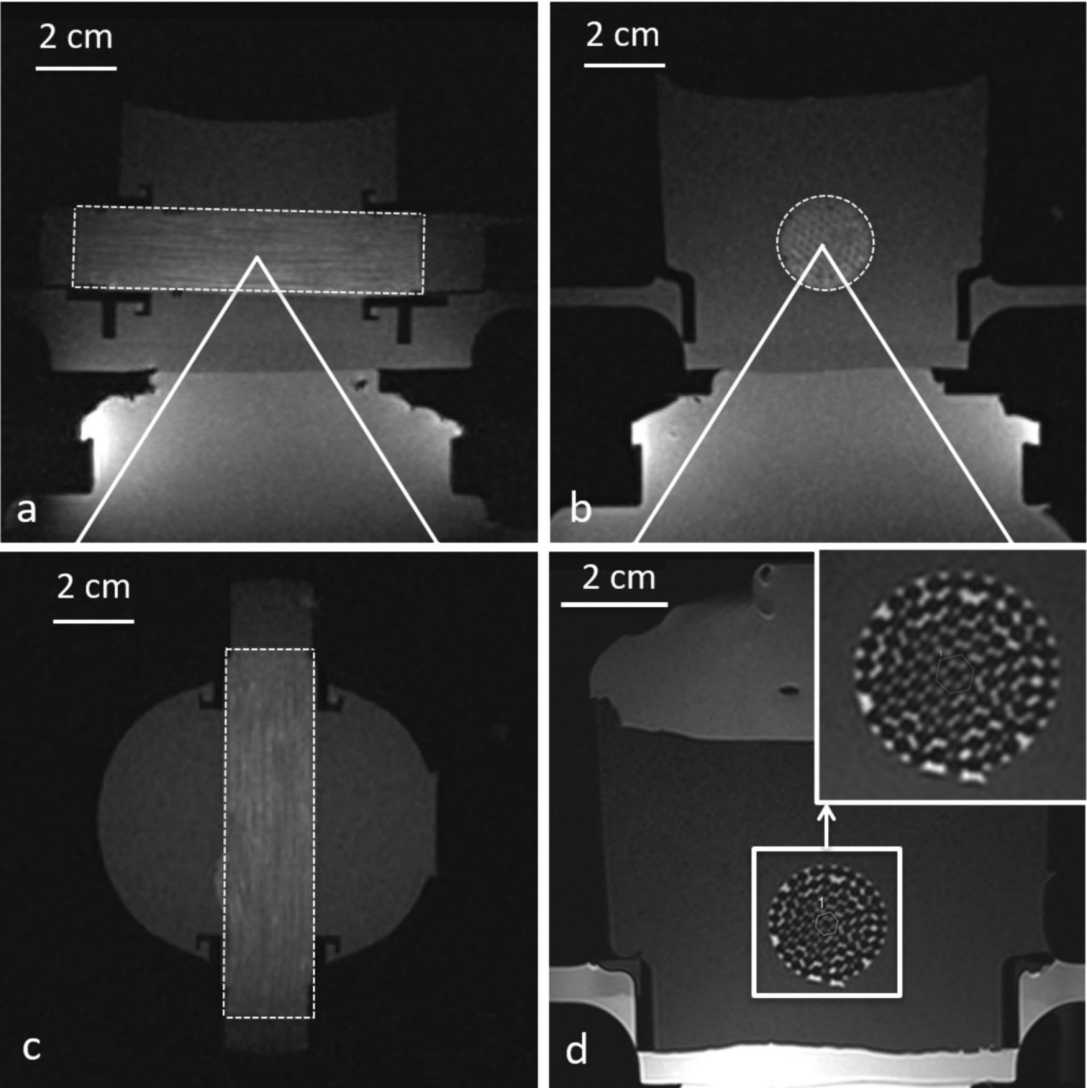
T1-VIBE high resolution MR images of the in vitro perfused model in three orthogonal planes (a) transverse, (b) sagittal and (c) coronal. T2-TSE high resolution MR image in the transverse (d), demonstrating the hexagonal packing with a gel-filling volume ratio of approximately 90% inside the cylindrical cavity of the matrix gel.
